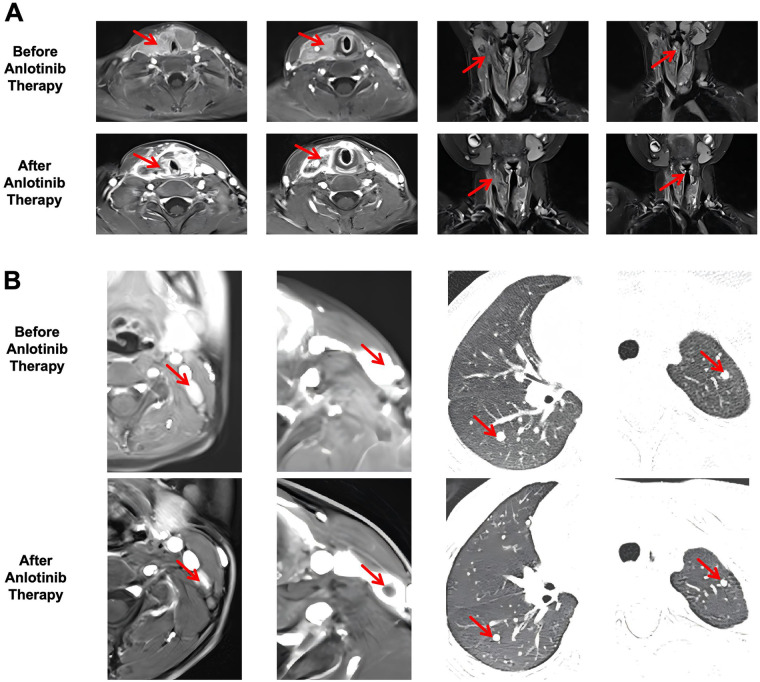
(A) imaging evaluation via MRI of the tumor's relationship with the right CCA, surrounding lesions, and tracheal compression before and after anlotinib treatment. From left to right: Thyroid tumor; Right CCA and surrounding lesions (sagittal view); Right CCA and surrounding lesions (coronal view); Tracheal compression. (B) Imaging evaluation of tumor size reduction via MRI and CT before and after anlotinib treatment. From left to right: Metastatic lymph node in left neck level II (MRI); Metastatic lymph node in left neck level IV (MRI); Metastatic lesion in right lung (CT); Metastatic lesion in left lung (CT). The key areas have been marked with red arrows.
